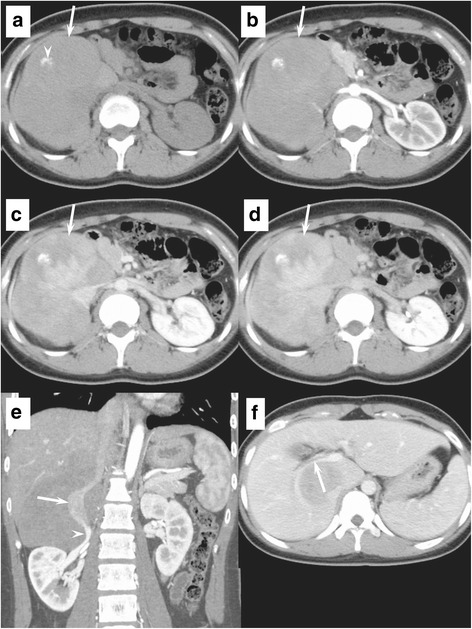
Abdominal computed tomography. a Unenhanced CT image shows a well-demarcated, expansile, soft tissue lesion (arrow) with focal calcification (arrow head) in the right peritoneum. b–d Dynamic contrast-enhanced CT images show delayed enhancement (arrows). e Coronal reformatted contrast-enhanced CT image shows dilatation of the IVC lumen circumferentially surrounded by the lesion (arrow) and stenosis of the right renal vein (arrow head). f Contrast-enhanced CT image shows the compressed right Glisson capsule (arrow)
